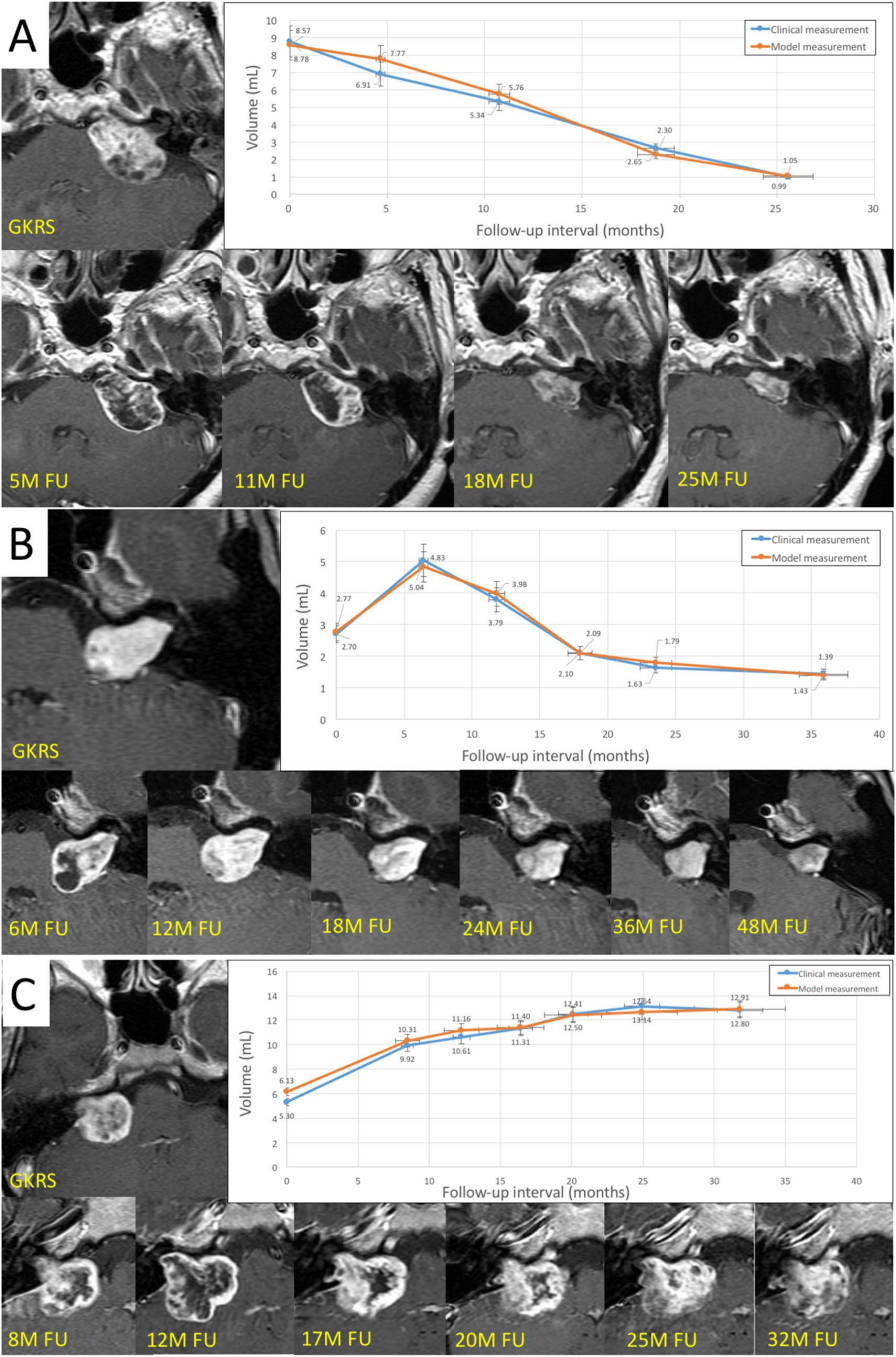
Case demonstration: (A) 46 y/o female VS patient who underwent GKRS and sequential MRI follow-up (follow-up MR images revealed positive response to radiation and ongoing tumor regression); (B) 53 y/o male VS patient who underwent GKRS and sequential MRI follow-up (follow-up MR images also showed positive response to radiation treatment with loss of MR imaging signal in center of tumor but transient enlargement of tumor); (C) 43-year-old female VS patient who underwent GKRS and sequential MRI follow-up (follow-up MR images obtained at 6 months after GKRS showed poor response to radiation treatment). A loss of central enhancement was observed in the early stage after GKRS; however, the tumor continued growing. At most follow-up time points, the differences between the predictions of AI models and clinical measurements were less than 10%. The trends of the tumor progression or regression predicted by the proposed AI model were remarkably accurate.
